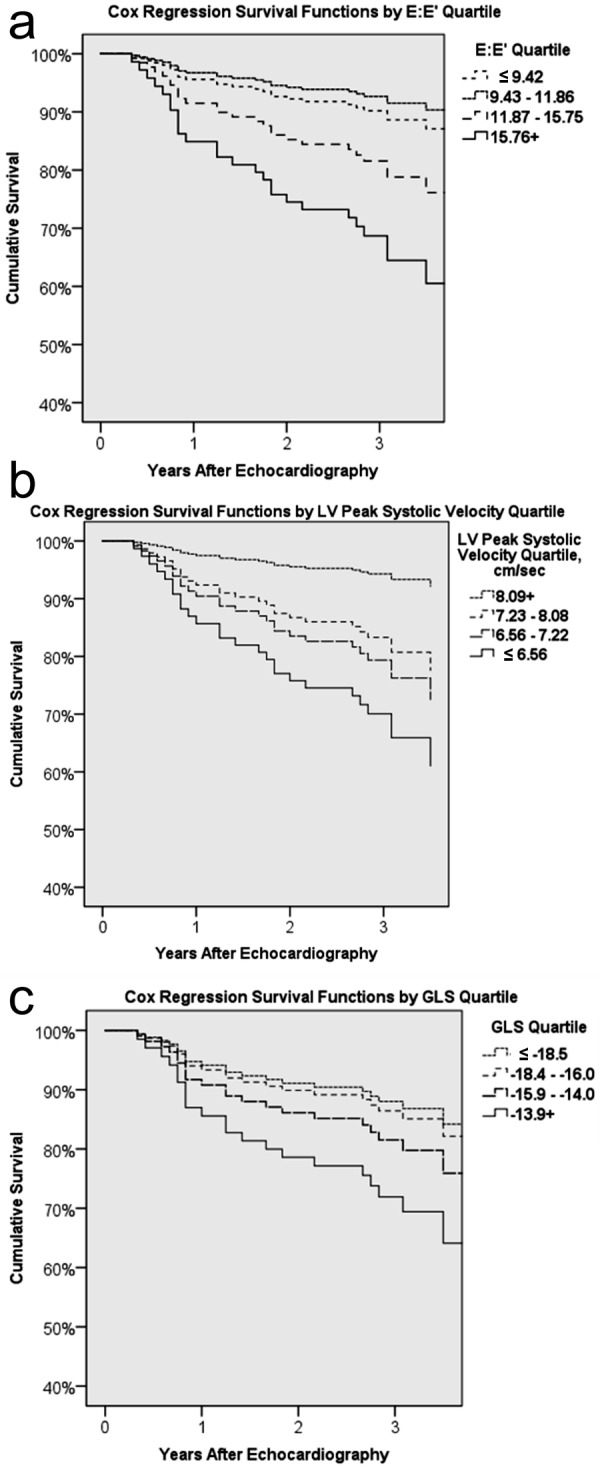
Figure 5a. Survival stratified by E:E’ quartiles. Cox regression survival functions stratified on the basis of E:E’ quartiles. E:E’ is an estimate of LV filling pressure and an indicator of the presence of advanced LV diastolic dysfunction. Values of E:E’ < 8 are considered normal whereas values equal to, or exceeding 15, are abnormal and consistent with elevated LV filling pressure. E:E’ is a strong predictor of survival in CKD-5D. Figure 5b. Cox regression survival functions stratified on the basis of peak LV systolic velocity quartiles. Peak LV systolic velocity is obtained using tissue Doppler echocardiographic imaging. Although the range of peak LV systolic velocity values is generally considered normal in this patient cohort, a significant relationship was seen between this measure and CAC burden and survival. Figure 5c. Survival functions by global longitudinal strain quartile from Cox regression analysis. Survival stratified by global longitudinal strain. Cox regression survival functions stratified on the basis of values of global longitudinal strain obtained from our CKD-5D patient cohort. GLS measures LV deformation during systole and is a sensitive marker of LV systolic function. More negative values are associated with normal LV systolic function. In our study, GLS was significantly associated with CAC burden and survival.
